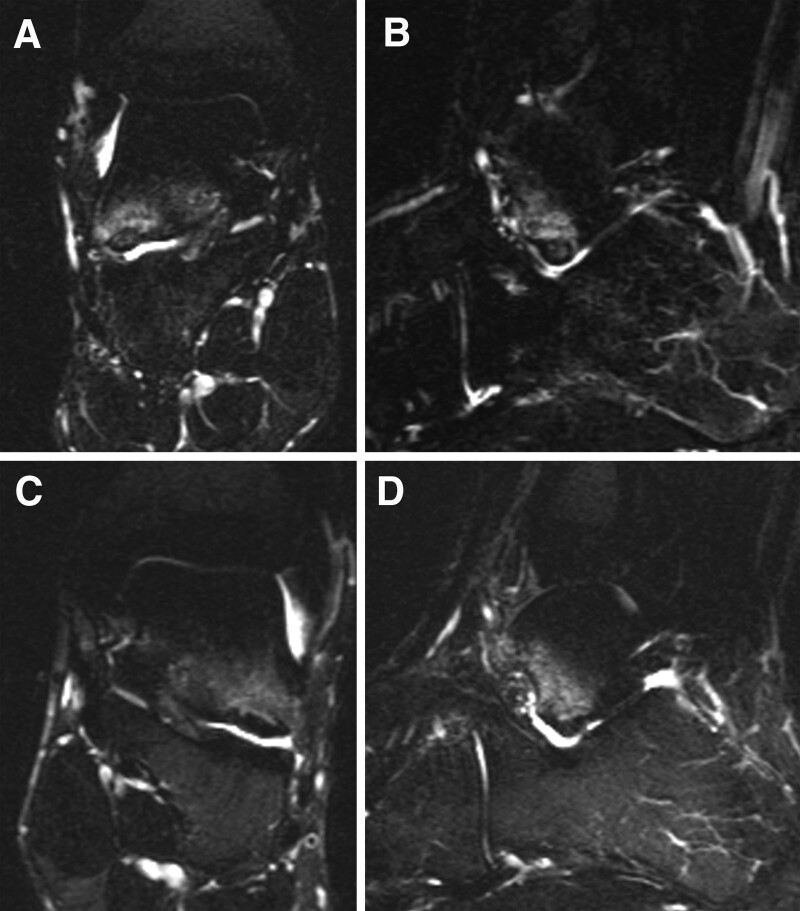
Preoperative magnetic resonance imaging of the right (A, B) and the left (C, D) feet reveal bone marrow edemas in the lateral process of the talus involving the subtalar joint; the lesion size was 6.9 × 6.8 mm in the right foot and 8.5 × 9.2 mm in the left foot (arrows).
